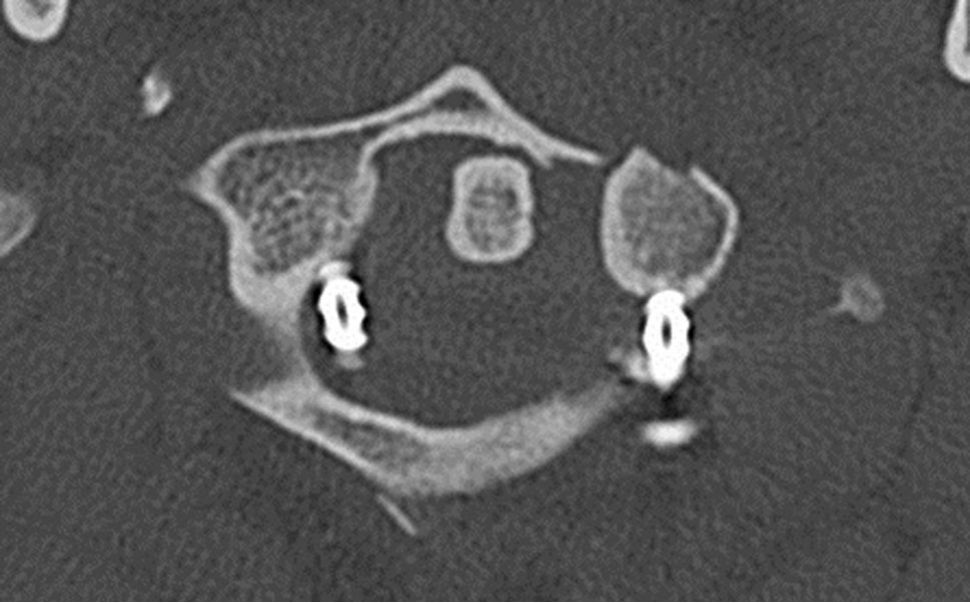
Transversal CT image: medial position of the right lateral mass screw in the spinal canal after surgery (patient 2)
